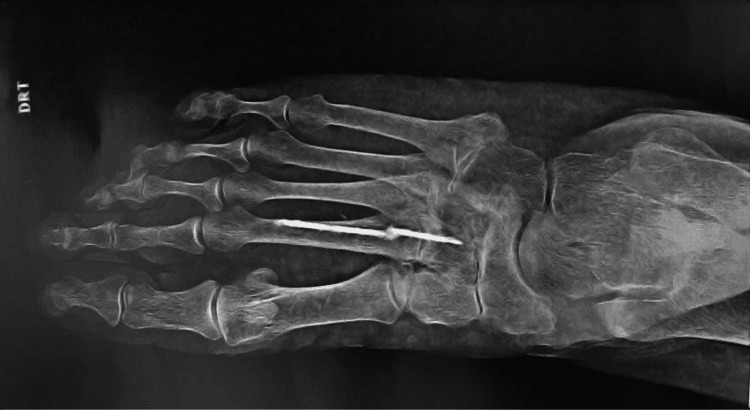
Diagnostic radiograph (anteroposterior incidence).
